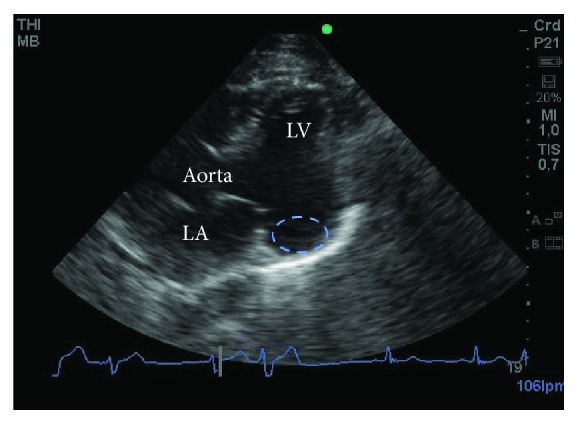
Transthoracic echocardiogram, parasternal long axis view. Notice the large submitral aneurysm (diameter, 3 cm) in the posterolateral basal-medial region of the left ventricle (encircled area). LA: left atrium; LV: left ventricle.
